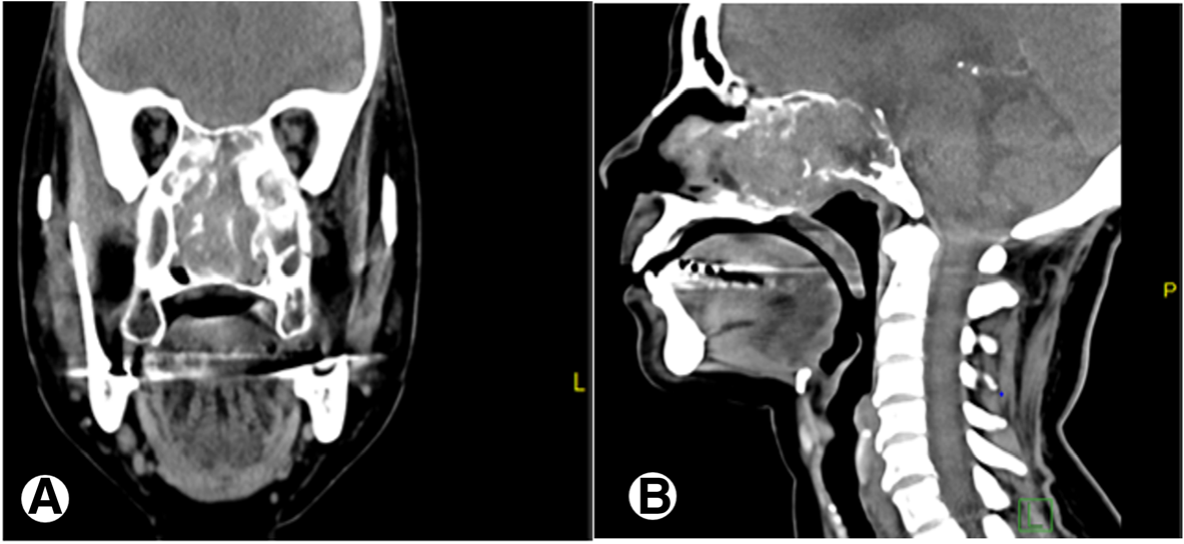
Head CT scan. Coronal head CT scan showing a destructive lesion within the paranasal sinuses extending to the nasal cavity. The epicenter of the mass is seen within the left ethmoid/posterior maxillary and sphenoid sinus (a) with extension posteriorly to involve the anterior superior border of the clivus (b)
